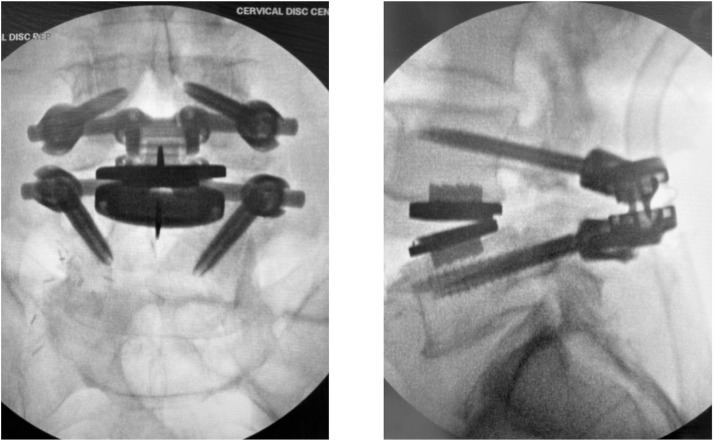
Anteroposterior (AP) and Lateral intraoperative radiographs after posterior phase Total Posterior Spine System (TOPS) implantation, forming the completed 360° motion-preserving construct.
